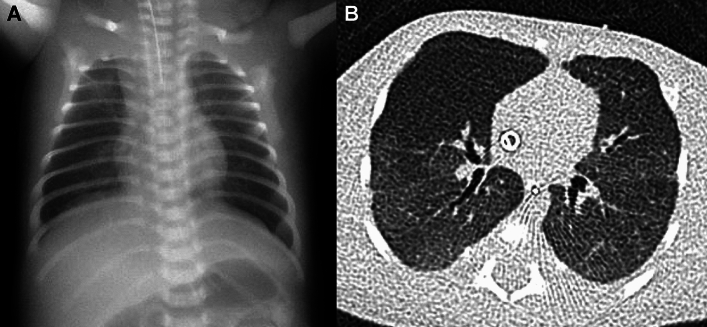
ACD-MPV. A neonate who presented with hypoxemia and hypercapnia immediately after birth, and later, severe pulmonary hypertension (less than 80% SpO_2_ despite NO inhalation). A CXR showed only mild hyperaeration and parahilar haziness in both lungs. B CT revealed increased lung densities that were mild but uniform
